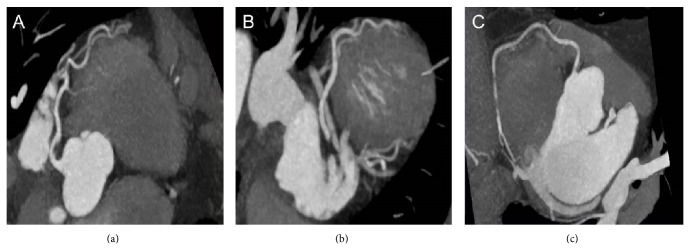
Coronary computed tomography showing absence of coronary artery calcium, with no evidence of arteriosclerotic disease.
